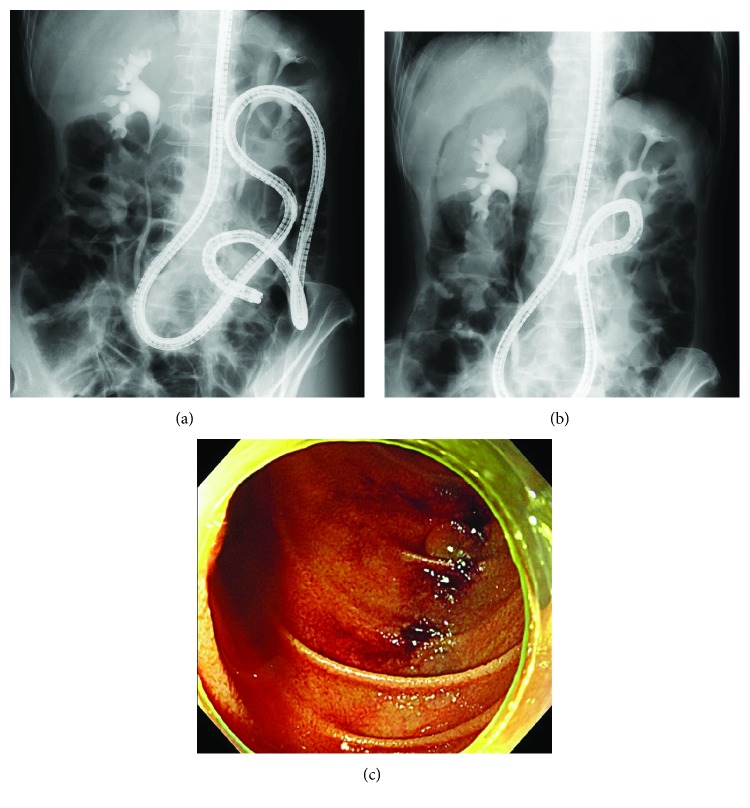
Perforation in a patient with peritoneal dissemination of gastric cancer. (a) An sSBE was inserted into the Treitz ligament, and a urethral stricture caused by peritoneal dissemination was found after contrast-enhanced CT. (b) When the intestine was shortened and an sSBE was inserted into the horizontal portion of the duodenum, free air was found around the right kidney. (c) When an sSBE advanced to the descending portion of the duodenum, 3 lacerations were found in the intestine contralateral to the papilla.
